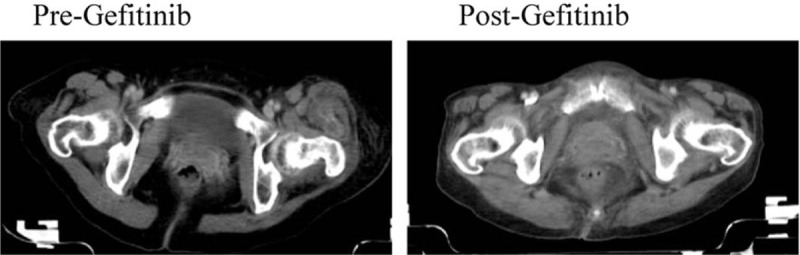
Pelvic CT images of the patient. Shrinkage of left femur metastases and decrease of soft tissue invasion after administration of gefitinib. CT = computed tomography.
